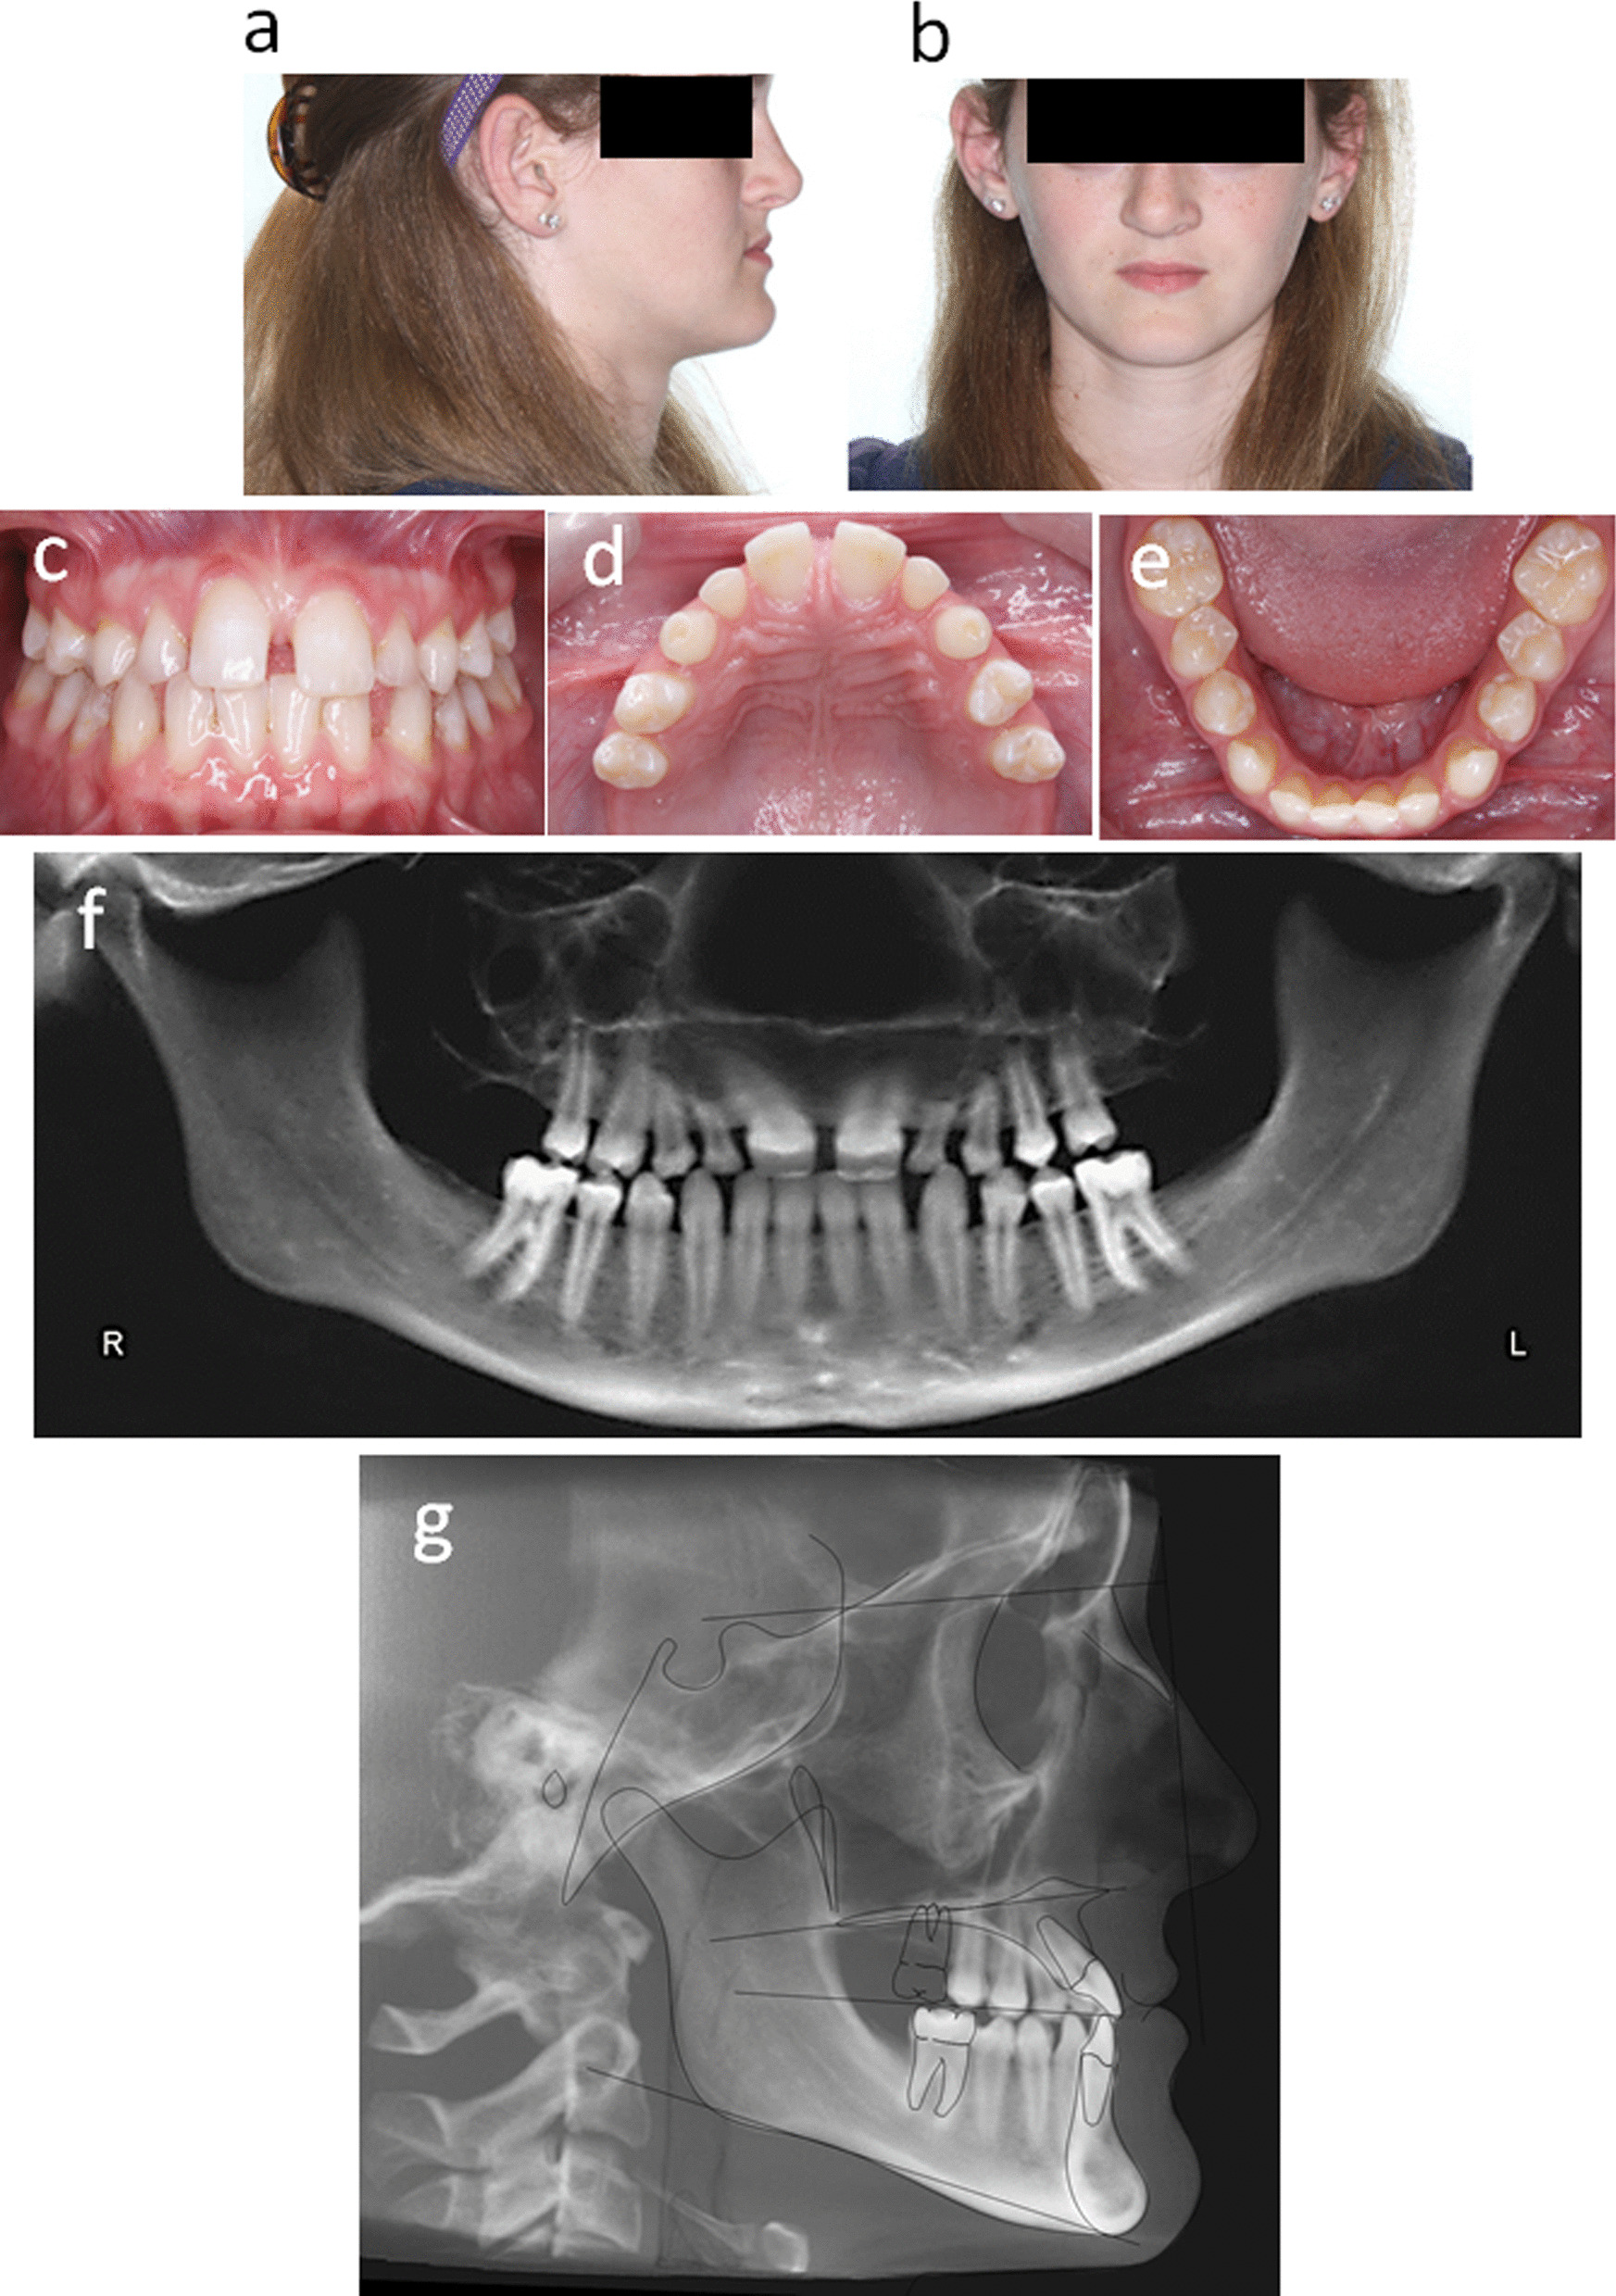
Initial examination before the treatment: a and b Extraoral photographs. c–e Intraoral photographs. f Panoramic radiograph. g The lateral cephalometric radiograph and tracing
